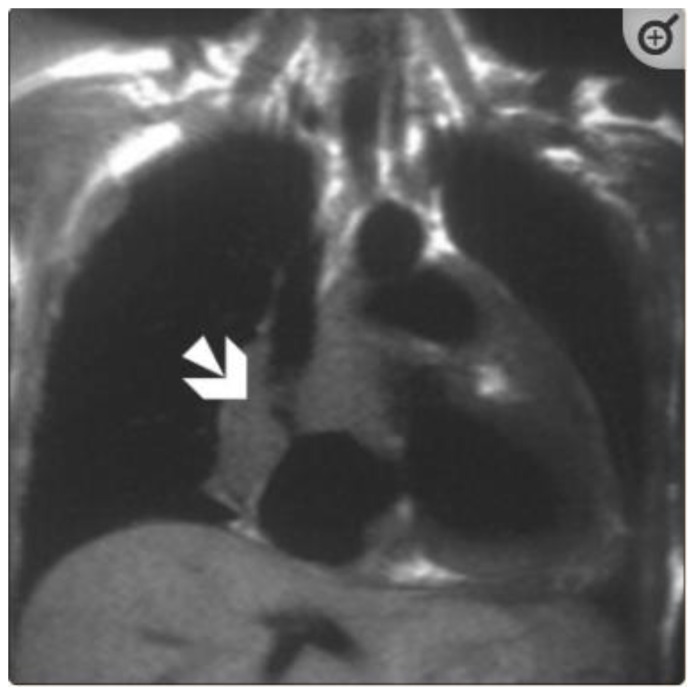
T2W dark-blood coronal MRI image shows diffuse myopericardial thickening. The thickening is hypointense on T2W images and is causing attenuation of the proximal SVC (arrow). The image was adapted from [53].
